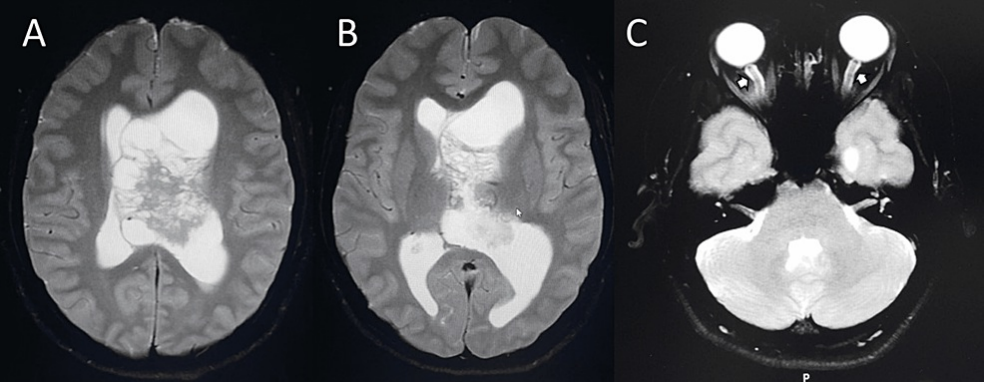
Magnetic resonance T2WI of the brain. (A) Axial plane at the lateral ventricle level; (B) Axial plane at the third ventricle level. The mass is isointense with a soap-bubble appearance and numerous cystic areas; (C) Mild distension of the subarachnoid space around bilateral optic nerves (arrows). T2WI: T2-weighted image
